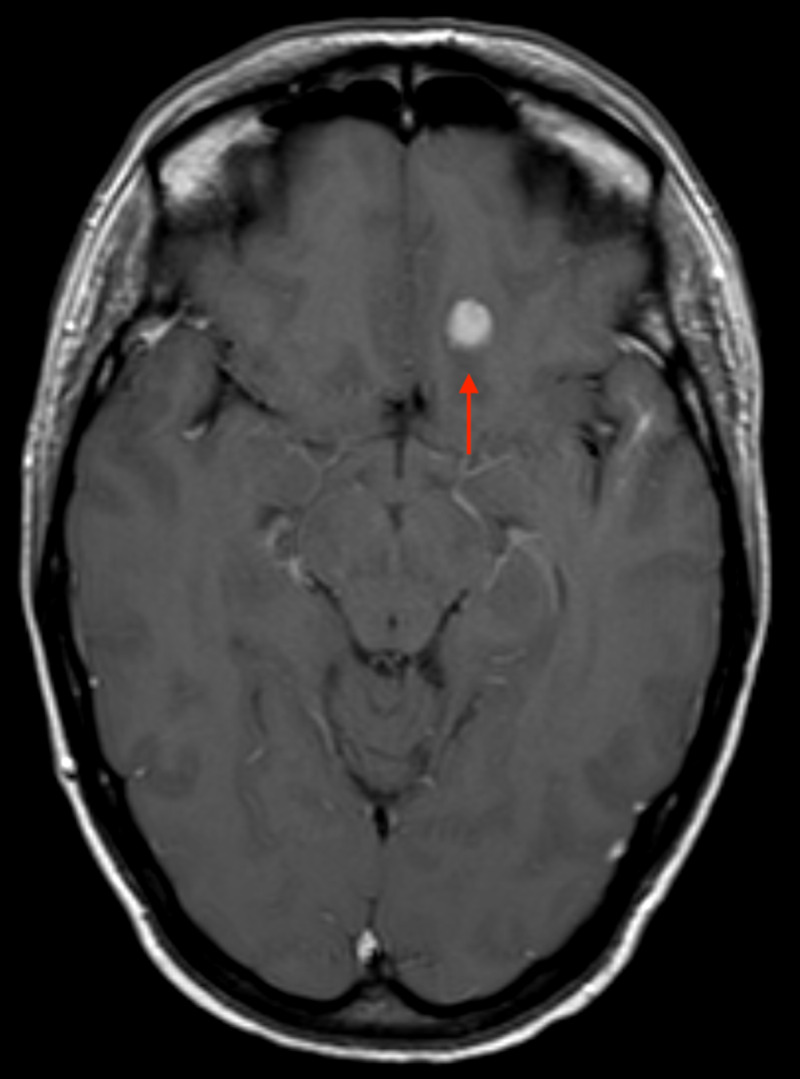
Axial T1-weighted MRI-contrast scan highlighting a solitary meningioma adjacent to the left-wing of the sphenoid.
